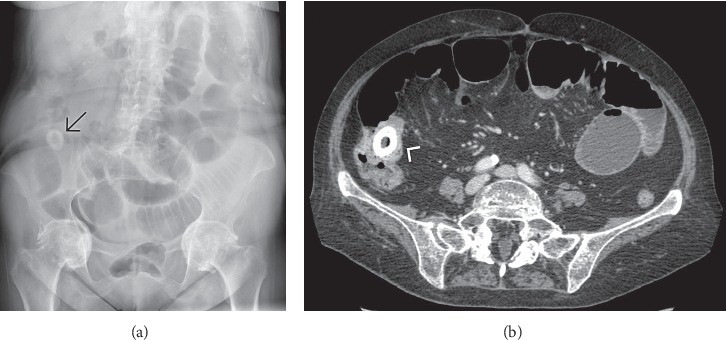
Supine abdominal radiograph (a) and contrast-enhanced computed tomography (b) in a case of gallstone ileus with a large calcified stone lodged at the level of the ileocecal valve, the most common site of bowel obstruction.
